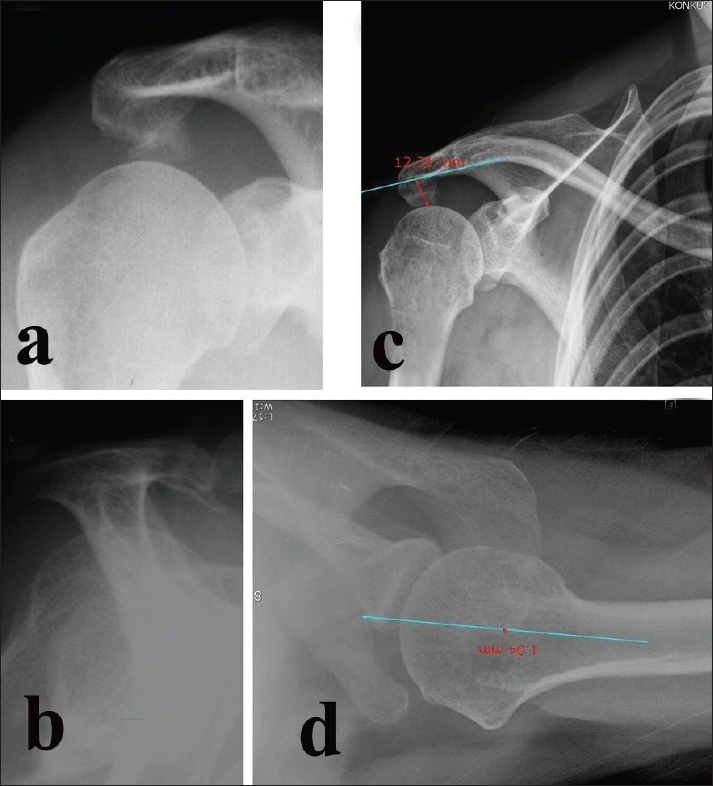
Showing (a) acromial spur in 30° caudal tilt view X-ray; (b) downward acromial spur in supraspinatus outlet view; (c) and (d) showing measurement of amount of acromial resection required in X-rays
